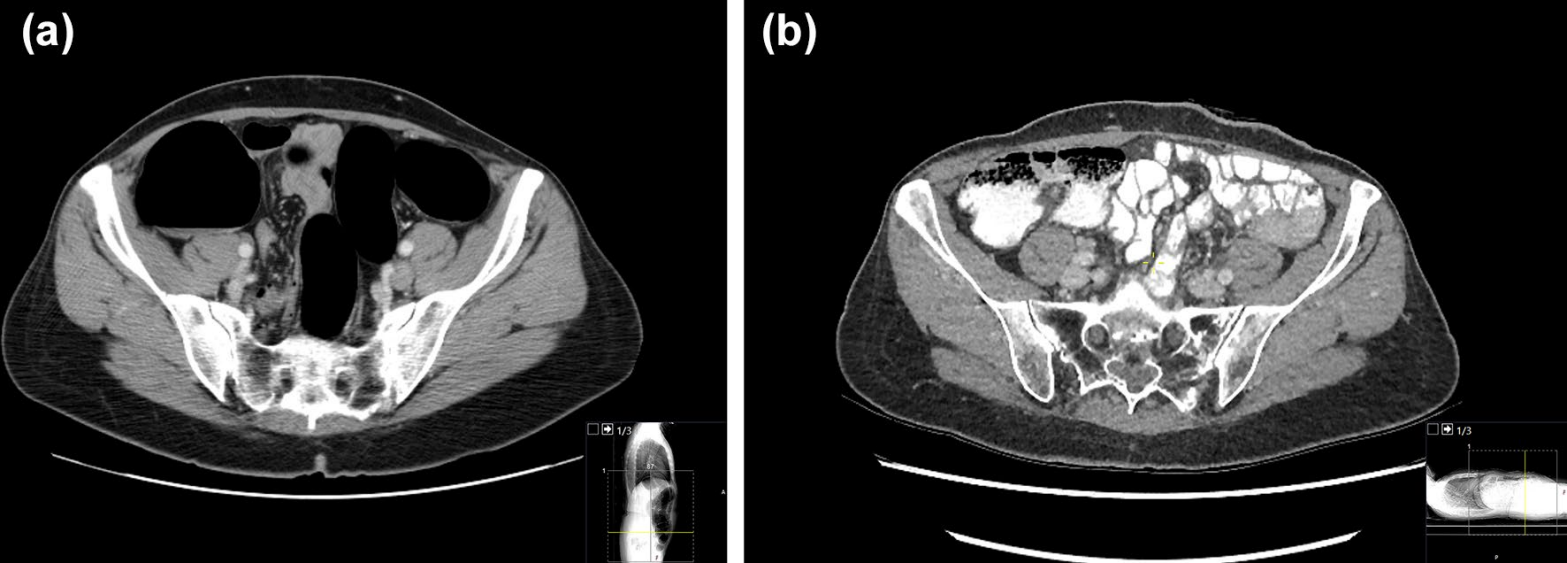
CT examinations of a patient with rectal cancer (a) pre- and b postoperative. The images are at the same level, caudal to the created stoma in the left rectus abdominis muscle (RAM). Preoperative findings of symmetric left and right RAM thickness and appearance. Postoperative marked asymmetry with normal appearance of the right RAM and a thin atrophic left RAM
